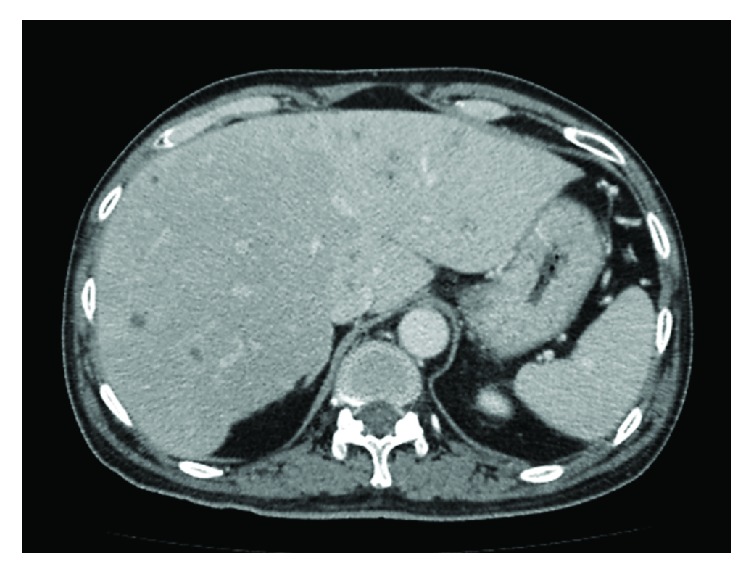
Axial computed tomography (CT) scan (portal venous phase) shows multiple small abscesses throughout both lobes of the liver. Transient hepatic attenuation differences (THAD) due to left portal vein thrombosis were well visualized. Note the normal-appearing spleen.
